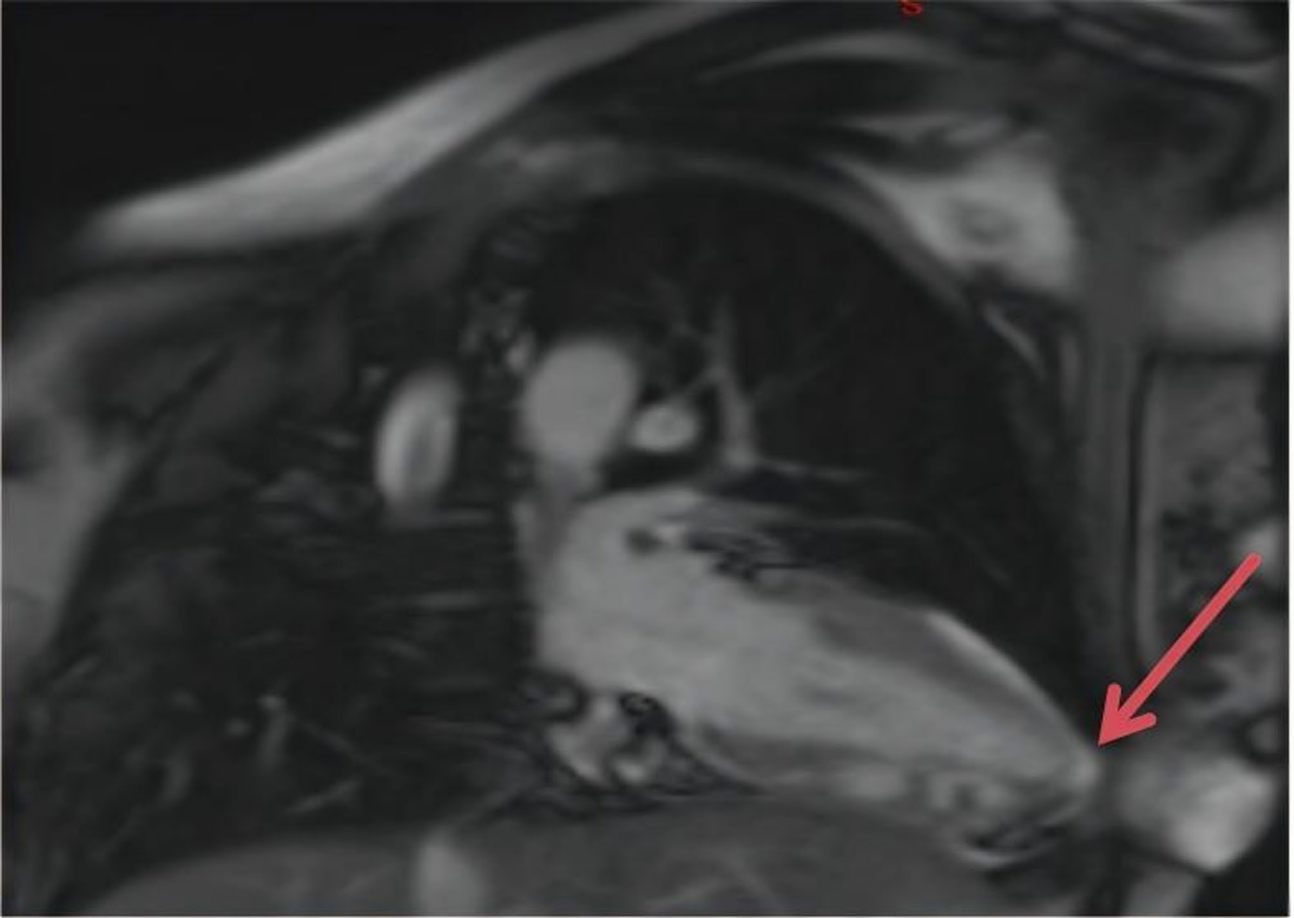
Cardiac MRI showing a ventricular septal dissecting aneurysm. An abnormal signal mass of approximately 16 mm × 42 mm in size partially communicated with the left ventricular cavity in the inferior wall of the interventricular septum
